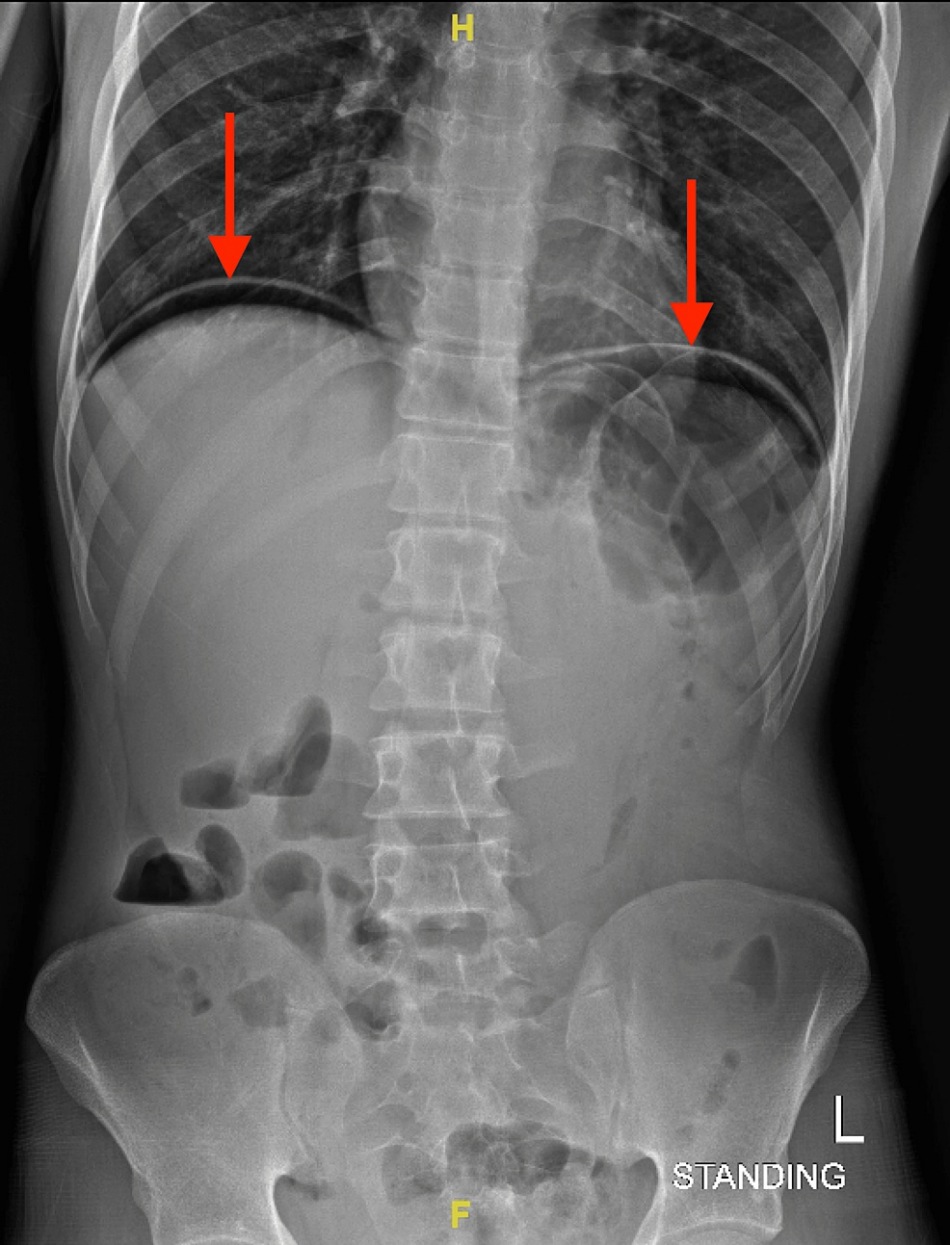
Erect abdominal x-ray. The red arrow shows bilateral air under the diaphragm.
